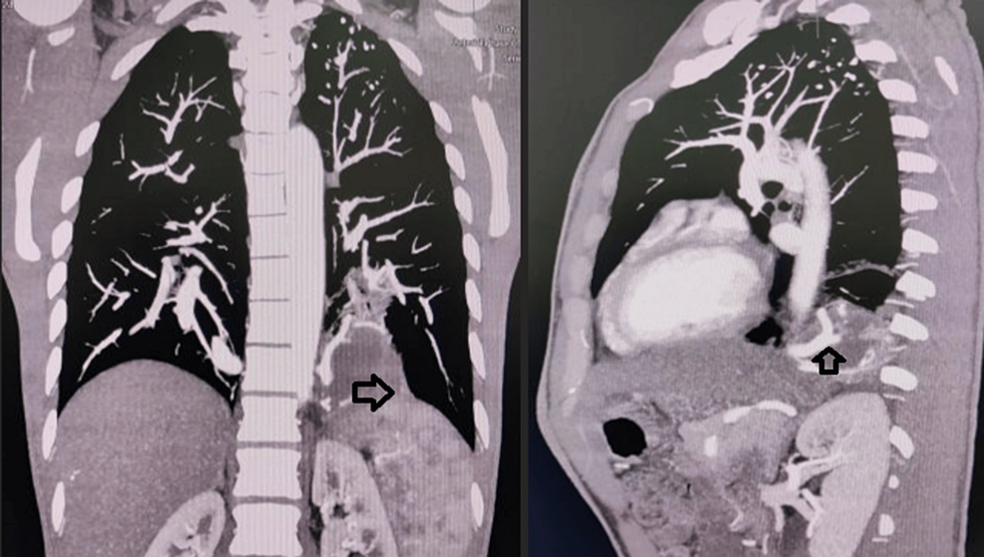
The coronal section of the CT angiogram shows the blood supply of sequestration tissue (arrow).
